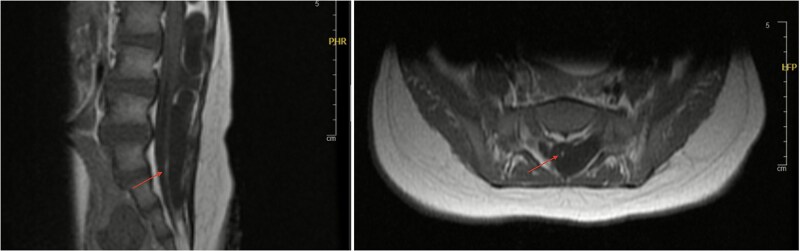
Axial and sagittal views of spinal MRI showing tethering of the cord at the level of S1 (arrows).
